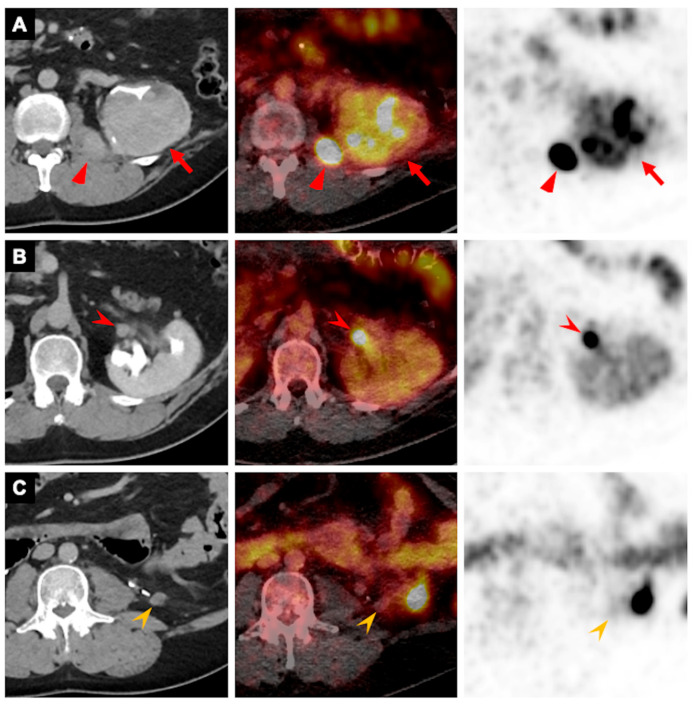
A 57-year-old man with a history of clear-cell renal cell carcinoma status post left partial nephrectomy ten years earlier presented with gross hematuria and was found to have suspected local recurrence. Axial portal venous phase CT images (left), ^89^Zr-girentuximab PET/CT fused images (middle), and PET images (right) demonstrate an enhancing left renal mass with heterogeneously intense uptake (arrows in (A)) and an enhancing, intensely avid nodule in the left psoas muscle (triangles in (A)). A perinephric soft-tissue nodule is seen anteriorly with intense uptake (arrowheads in (B)), suspicious for tumor deposit. An additional inferior nodule shows no uptake (arrowheads in (C)) and is unchanged compared with the postoperative CT obtained nine years earlier, consistent with benign postoperative changes. The patient subsequently underwent left radical nephrectomy with psoas mass resection. Pathology confirmed recurrent clear-cell renal cell carcinoma with psoas muscle metastasis.
